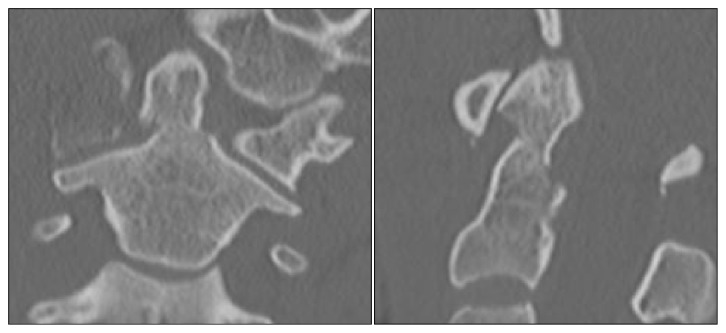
CT images in coronal (left panel) and sagittal (right panel) reconstructions, showing a 4mm displaced type II odontoid fracture.
